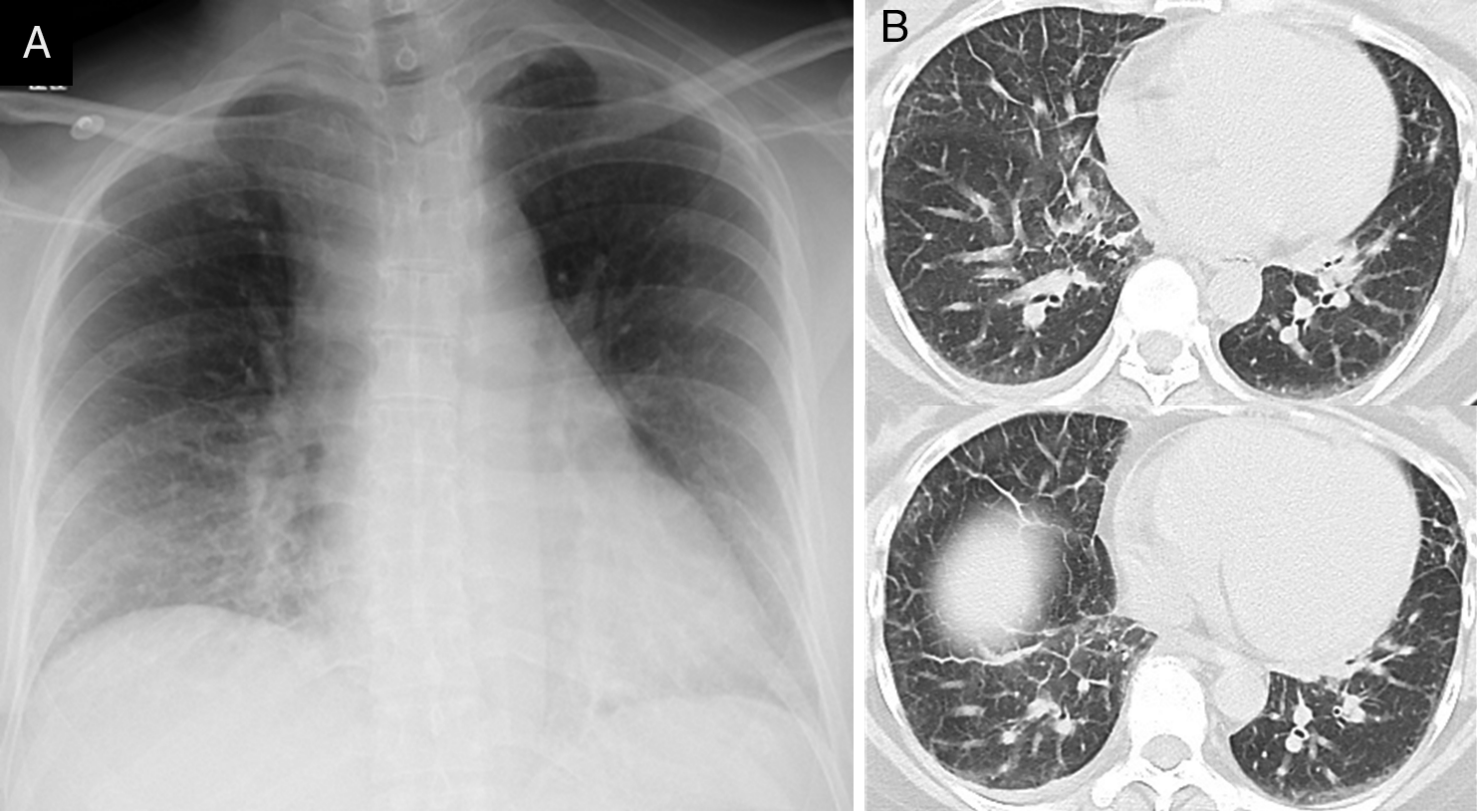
(A) Chest radiograph obtained on admission showing faint infiltration in the lower lung fields. (B) Computed tomography scan showing interlobular septal thickening and peribronchovascular interstitial thickening.
